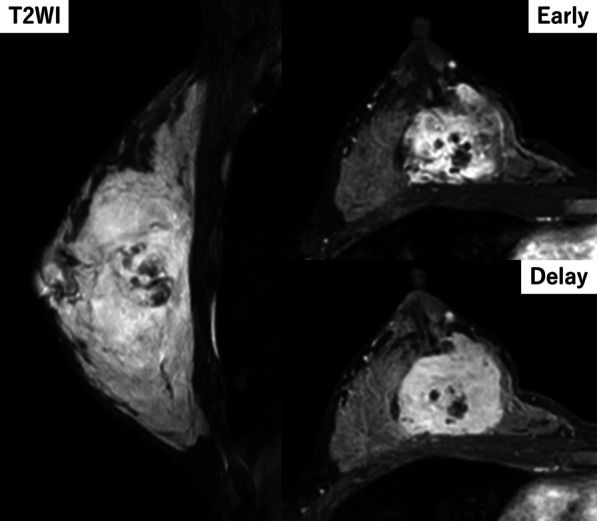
MRI findings. High T2 signal intensity mass occupied the entire right breast. It demonstrated rapid enhancement post-contrast administration with progressive and prolonged enhancement in the delay phase
